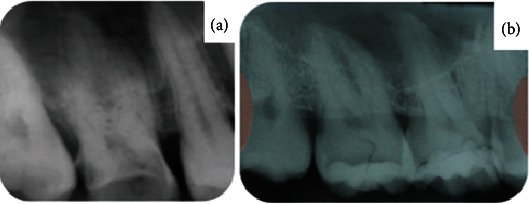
Periapical radiographs of tooth 16 in (a) 2016 and (b) 2023.
